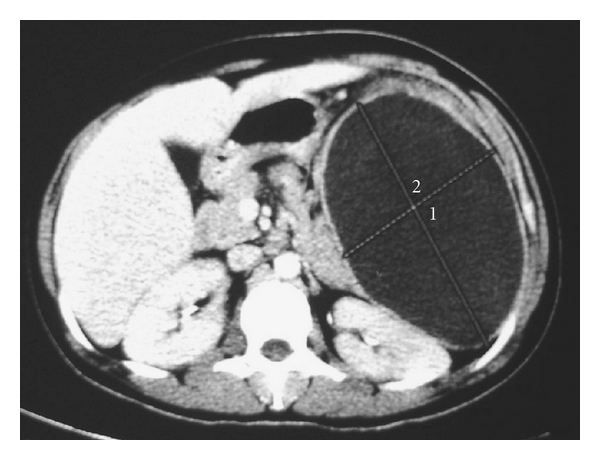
Abdominal CT scan showing a large splenic abscess occupying the whole spleen in a child with sickle cell anemia.
